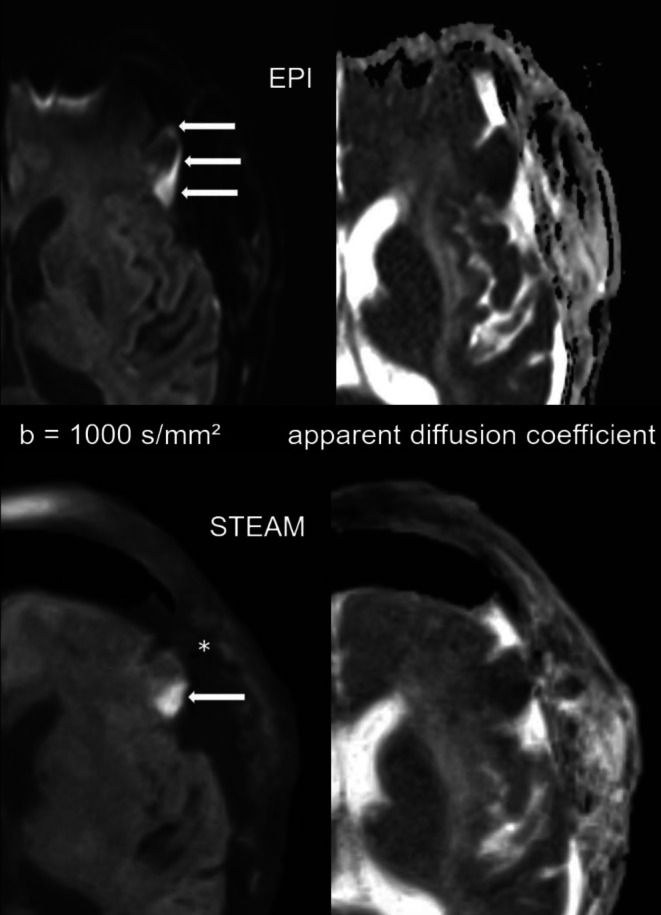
77-year-old patient. Postoperative diffusion-weighted images with b = 1000 s/mm^2^ (*left*) and apparent diffusion coefficient maps (*right*) obtained by EPI DWI (*top*) and STEAM DWI (*bottom*) 71 h after resection of a left temporal astrocytoma. In EPI DWI the border between infarct area (*arrows*), cerebrospinal fluid and air is not clear due to geometric distortion, whereas in STEAM DWI the infarct area (*arrow*) is sharply outlined and even small air bubbles (*asterisk*) can be detected
